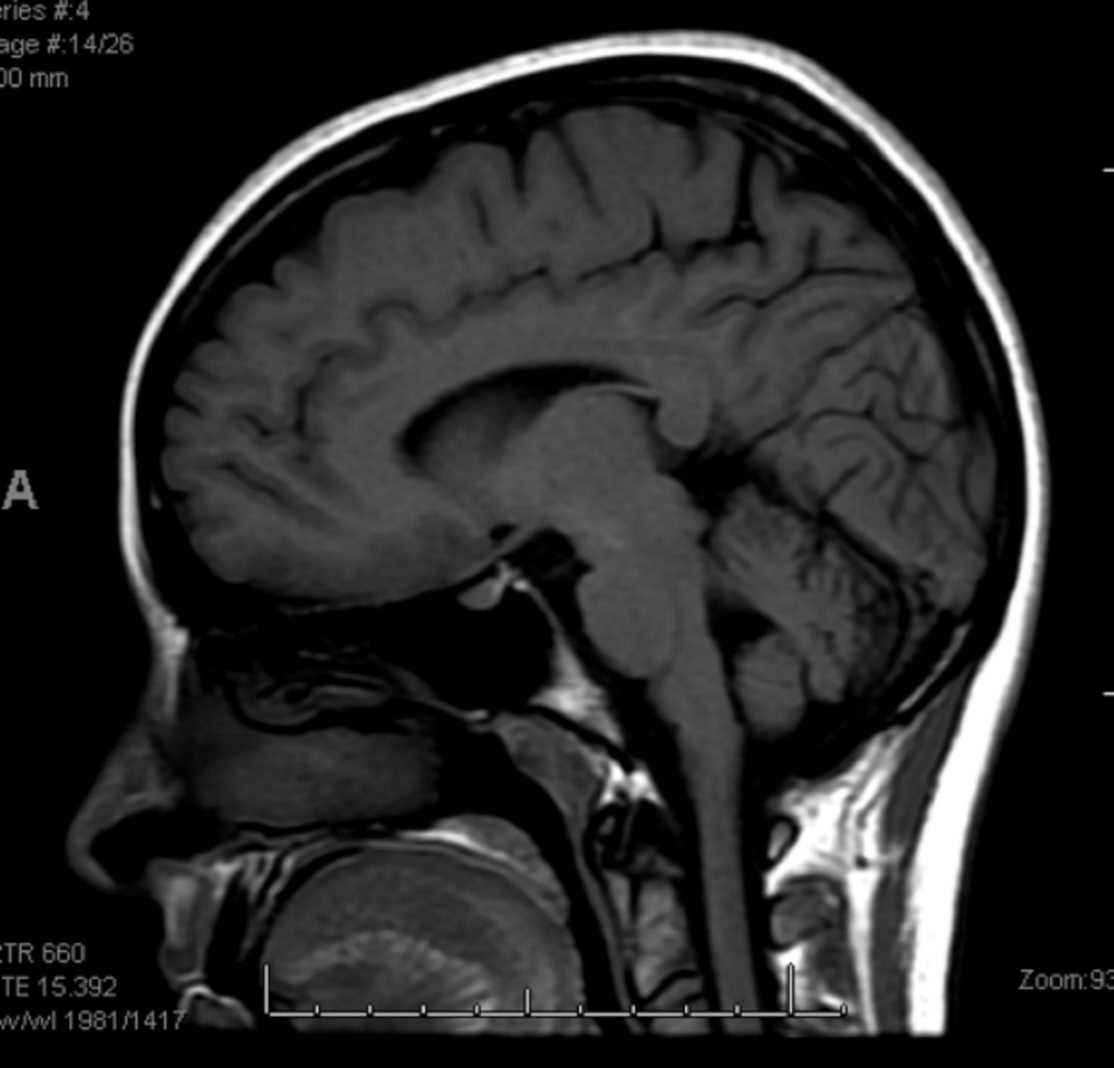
Repeat MRI brain sagittal section did not show any enhancing/hyperintense lesions.
